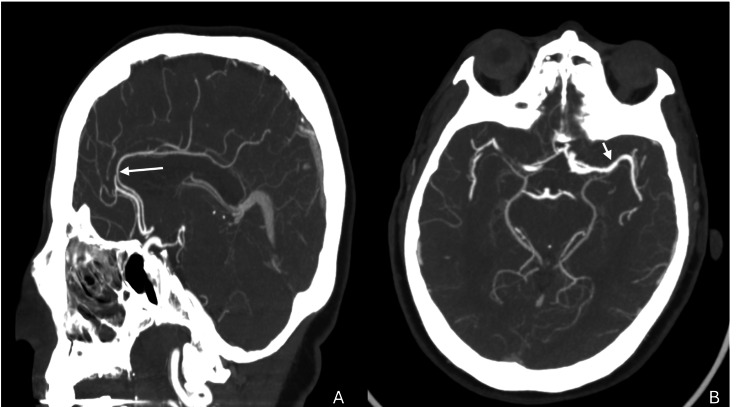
CT angiography (CTA) demonstrating distal left anterior cerebral artery occlusion. (A) Sagittal view demonstrating an occlusive thrombus in the distal left A2 segment (arrow). (B) Axial view demonstrating preserved patency of the left middle cerebral artery (arrow).
